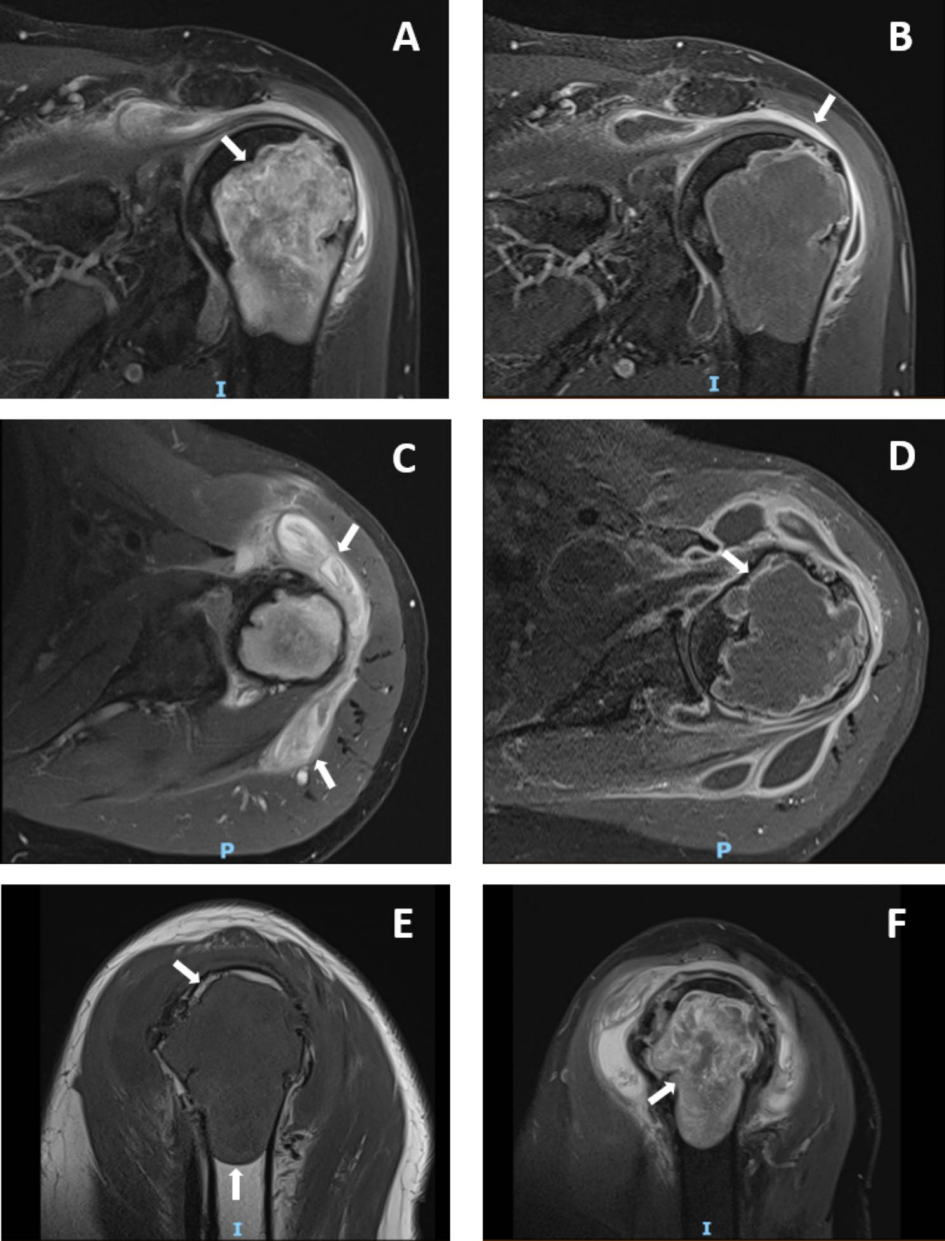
Magnetic resonance imaging (MRI) of the left shoulder in the RA patient. Coronal T2-weighted MR image (A) shows a high signal intensity, heterogeneous and well-defined intramedullary bone lesion with sclerotic border (white arrow). Coronal T1 contrast-enhanced (B) and Axial T2-weighted MR images (C), both shows a subacromial-subdeltoid bursa distension (white arrows). Axial T1 contrast-enhanced MR image (D) shows a high signal intensity, heterogeneous lesion (geode), with peripheral synovium enhancement (white arrows). Sagittal T1 MR image (E) show an iso signal intensity, intramedullary lesion, with well-defined contours surrounded by a sclerotic border (white arrow) in the proximal metaepiphysis region of the humerus. Sagittal T2-weighted MR image (F) shows a high signal intensity, intramedullary lesion, representative of a geode, fulfilled with synovium (white arrow)
